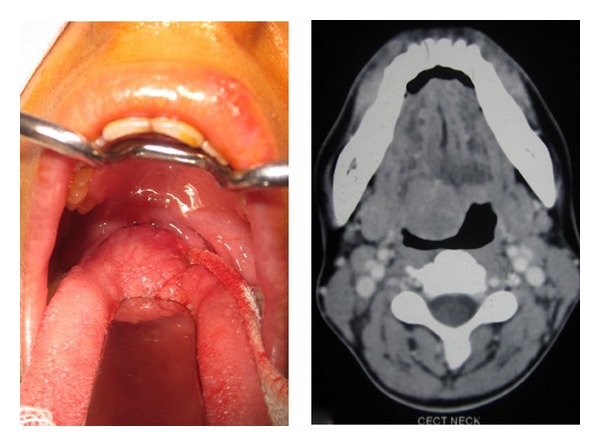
Figure showing the tumor in the base of tongue and the preoperative CT scan.
